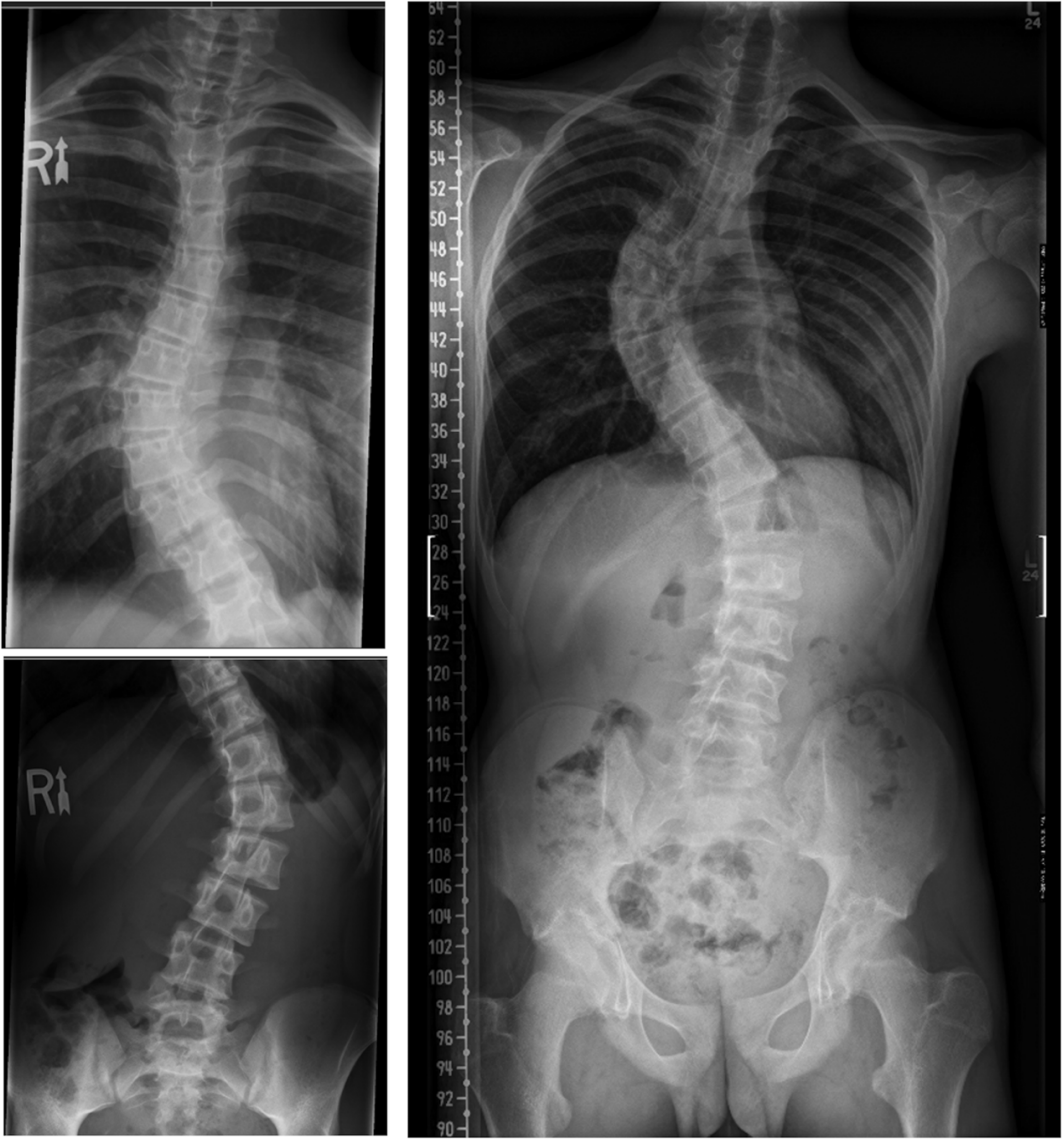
Radiographs of adolescent idiopathic scoliosis in 12-year-old patient. (Left) Radiographs performed in a community centre. Cobb angle of a single curve reported as 19°C. No Risser stage reported. (Right) Repeat radiograph during consultation for same patient. Two curves identified: thoracic and lumbar curve measuring 46°C and 59°C, respectively. Risser stage of 3 reported.
